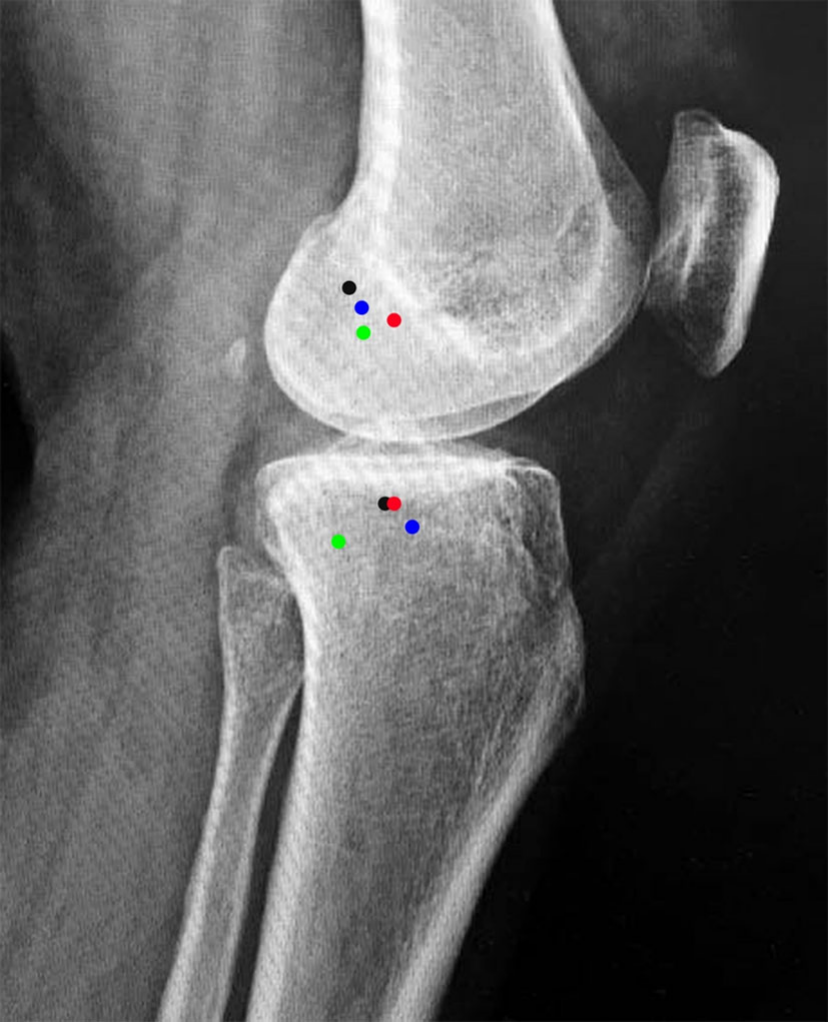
Radiographic landmarks. Lateral knee radiograph approximately showing the landmarks described by Helito et al. (*red*), Kennedy et al. (*black*), Rezansoff et al. (*green*) and Heckmann et al. (*blue*) [28, 32, 42, 72] (color figure online)
